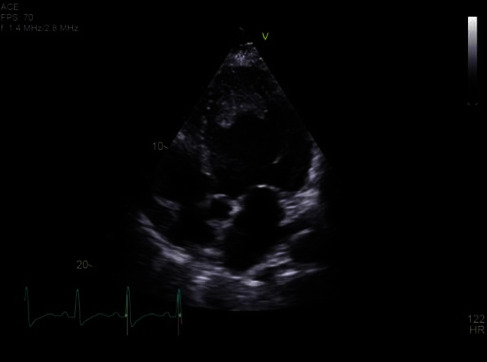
Transthoracic echocardiogram showed globally impaired left ventricular systolic function and a large left ventricular thrombus.
